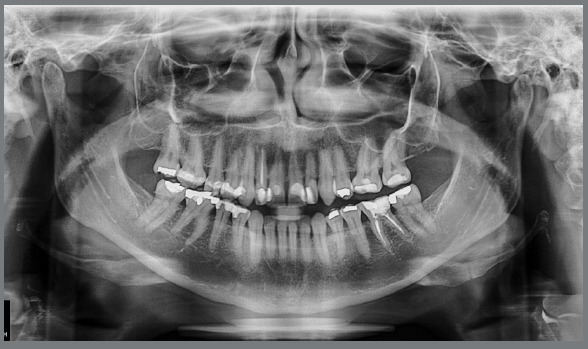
Baseline panoramic radiograph.
